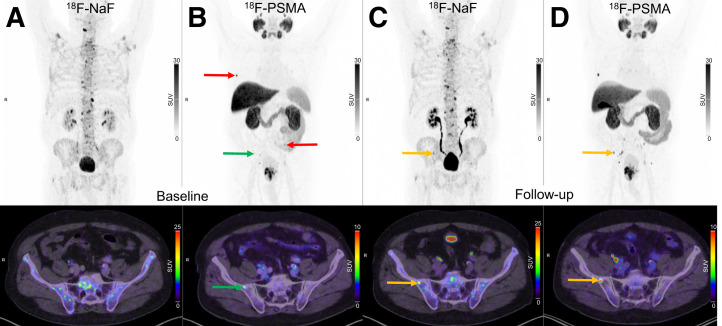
Patient with discordant findings on baseline ^18^F-NaF and ^18^F-PSMA PET/CT. (A) Maximum-intensity projection and axial projection of ^18^F-NaF PET/CT scan with no evidence of bone metastases. (B) ^18^F-PSMA PET/CT scan showing 2 lesions, one in left ilium and another in right costa 6, which were suspected of being bone metastases (red arrows). Third faint lesion in right ilium was not interpreted as bone metastasis (green arrows). Because of absence of metastatic lesions on ^18^F-NaF PET/CT (conventional scan), patient was observed, without treatment. (C and D) Follow-up scans conducted 6 months later revealed detectable uptake in lesion in right ilium on ^18^F-NaF PET/CT and increasing uptake on ^18^F-PSMA PET/CT (orange arrows). There are multiple ^18^F-PSMA-avid lymph nodes on follow-up scan.
